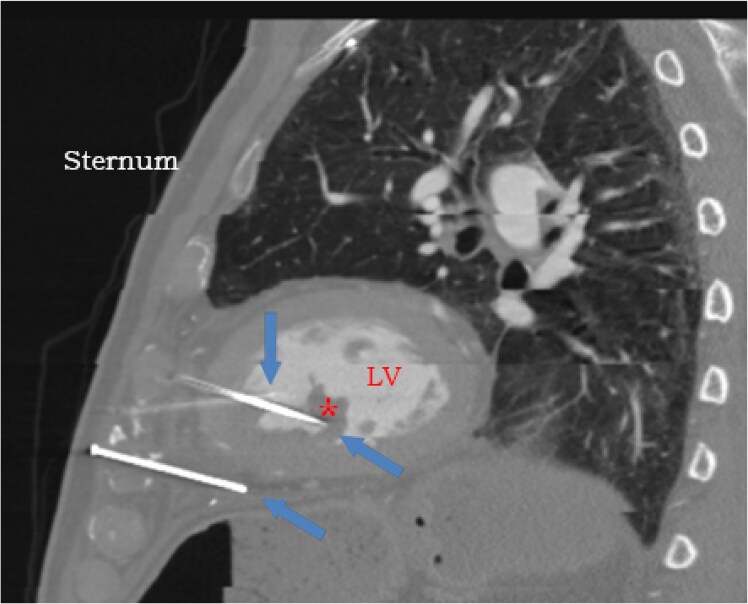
Computed tomographic angiography of the heart showing three nails penetrating thoracic cavity, two of which penetrate through the heart. The left ventricular (LV) cavity is noted. The tip of the first nail (top blue arrow) terminates in the sub-endocardium of the mid interventricular septum. The tip of second nail (middle blue arrow) appears to terminate in the posteromedial papillary muscle (red asterisk). The third nail (bottom blue arrow) terminates adjacent to the inferior wall of the left ventricular myocardium.
